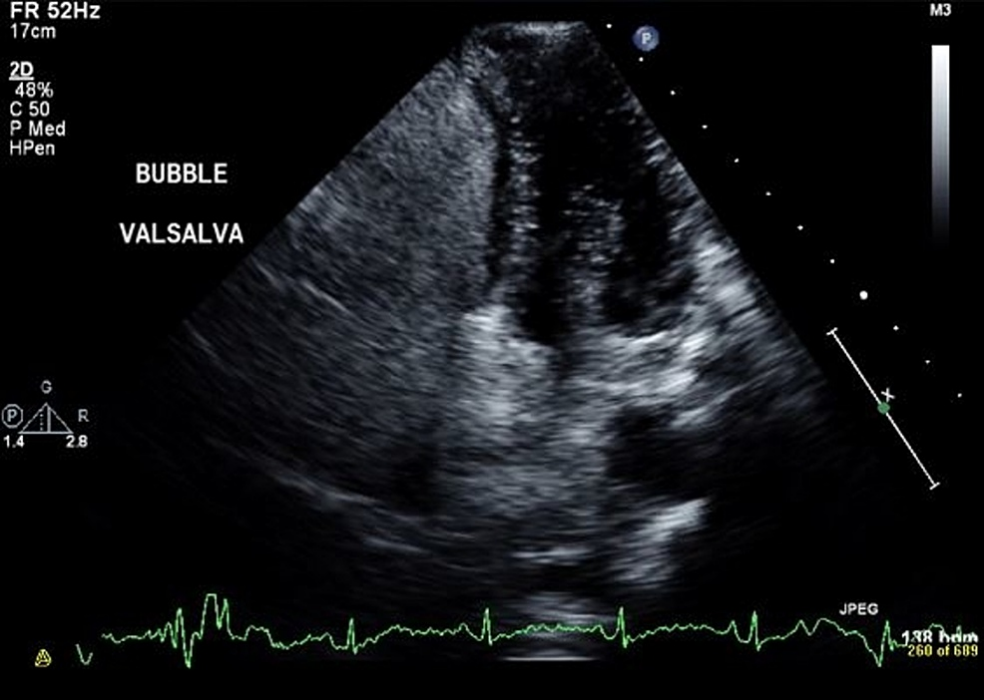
Transthoracic four-chamber view with bubble study demonstrating right to left shunt during Valsalva maneuver.
